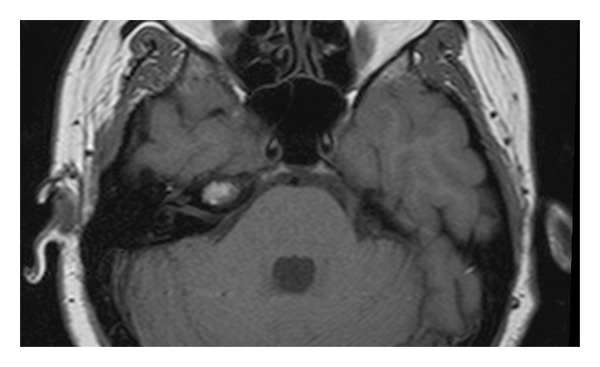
Axial T1-weighted image shows a hyperintense lesion in the right petrous apex.
